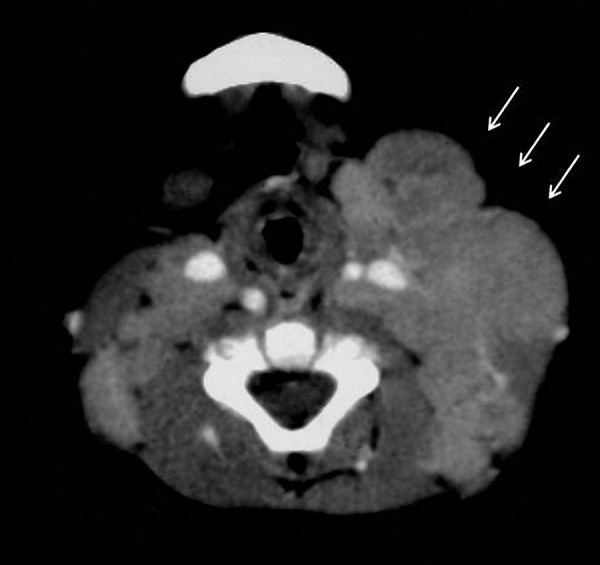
Radiologic findings in the patient at the age of 9 months. Axial contrast-enhanced computed tomography shows left cervical adenopathy (arrows).
